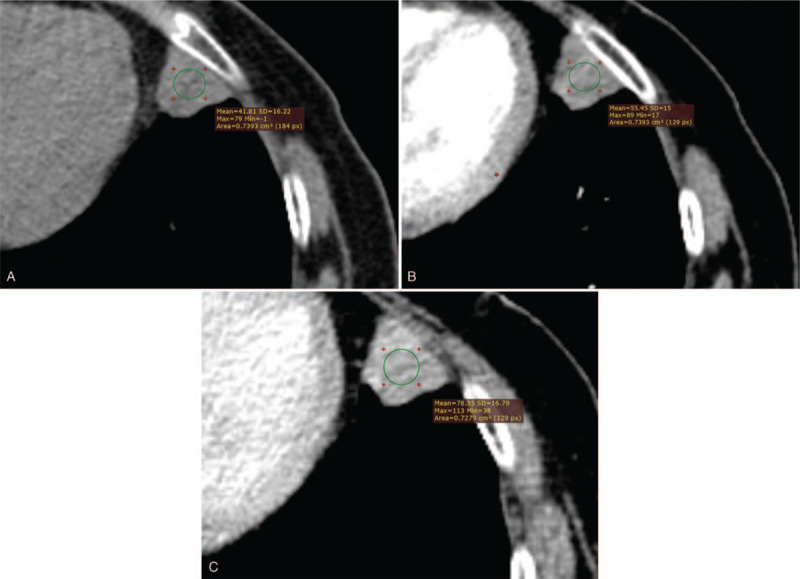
Enhanced thoracic CT images in a 65-year-old woman with PLELC. Soft-tissue window (A, B, C) shows obvious delayed enhancement of the nodule (red circle). CT = computed tomography, PLELC = pulmonary lymphoepithelioma-like carcinoma.
